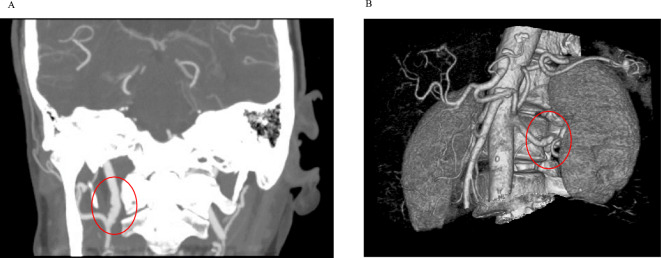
CT findings suggestive of FMD in the clinical case. A Internal carotid artery beading and aneurysms displayed on CT intracranial angiogram. B Left renal artery beading displayed in a 3-dimensional reconstruction model of the CT image. *CT* computed tomography, *FMD* fibromuscular dysplasia
